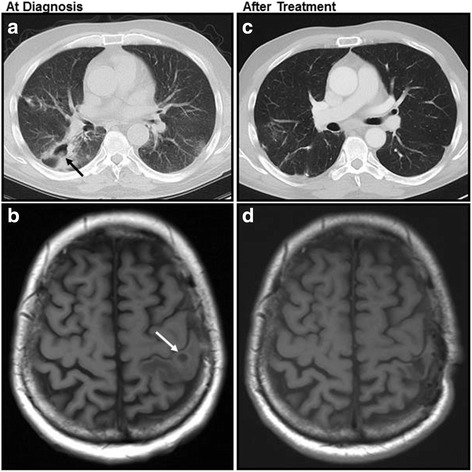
CT-scan of the lungs and MRI of the brain in early stage of disease (a, b) and after treatment (c, d), showing bilateral infiltrates and cavitations (a, black arrow) and cerebral abscess (b, white arrow). After therapy, only residual defects can be observed (c, d)
